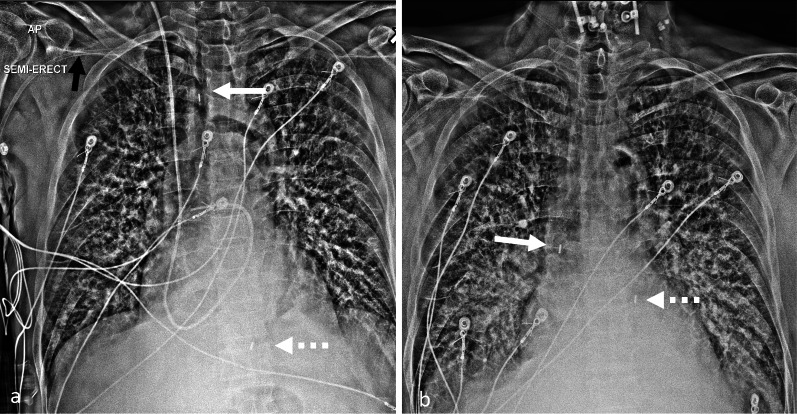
Malposition of axillary IABP. AP chest radiograph with contrast limited adaptive histone equalization (CLAHE) filter demonstrating an axillary approach IABP (a, b). The IABP is malpositioned with the upper marker in the brachiocephalic artery (white arrow on image a). The black arrow indicates the location of the sheath. The inflated balloon is seen in the aortic arch and upper descending aorta. The inferior marker is within the descending aorta (dashed arrow). b AP chest radiograph after attempted advancement of the IABP, the superior marker is malpositioned and is now in the ascending aorta (white arrow). The inferior marker is in the descending aorta (dashed arrow). (IABP = Intra-aortic balloon pump, CLAHE = Contrast limited adaptive histone equalization)
